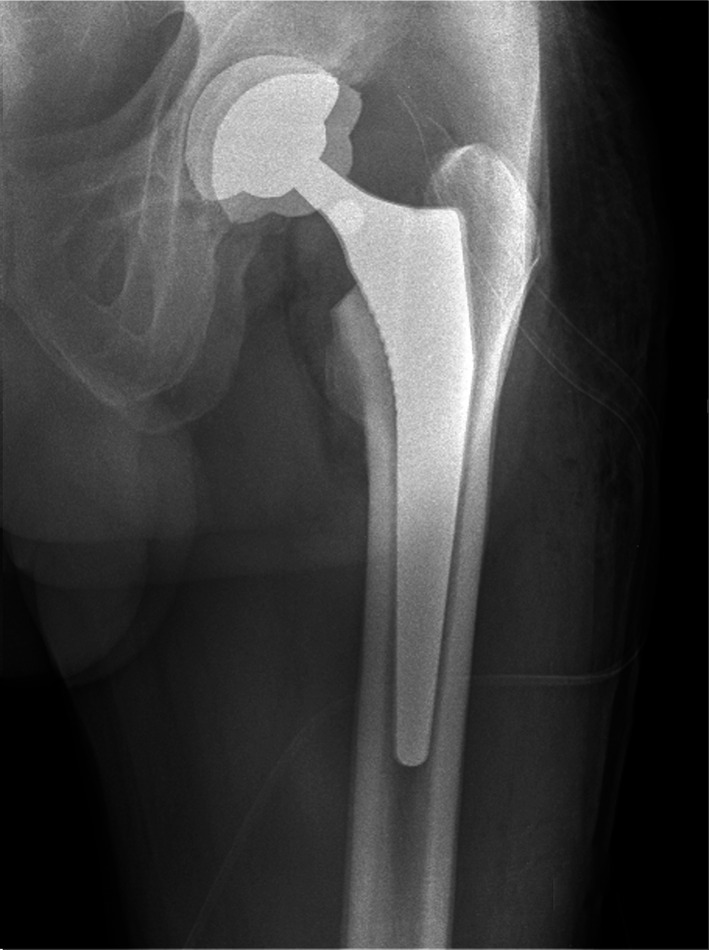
Superposition x-ray femoral stem between index and revision surgery, shortening the leg
